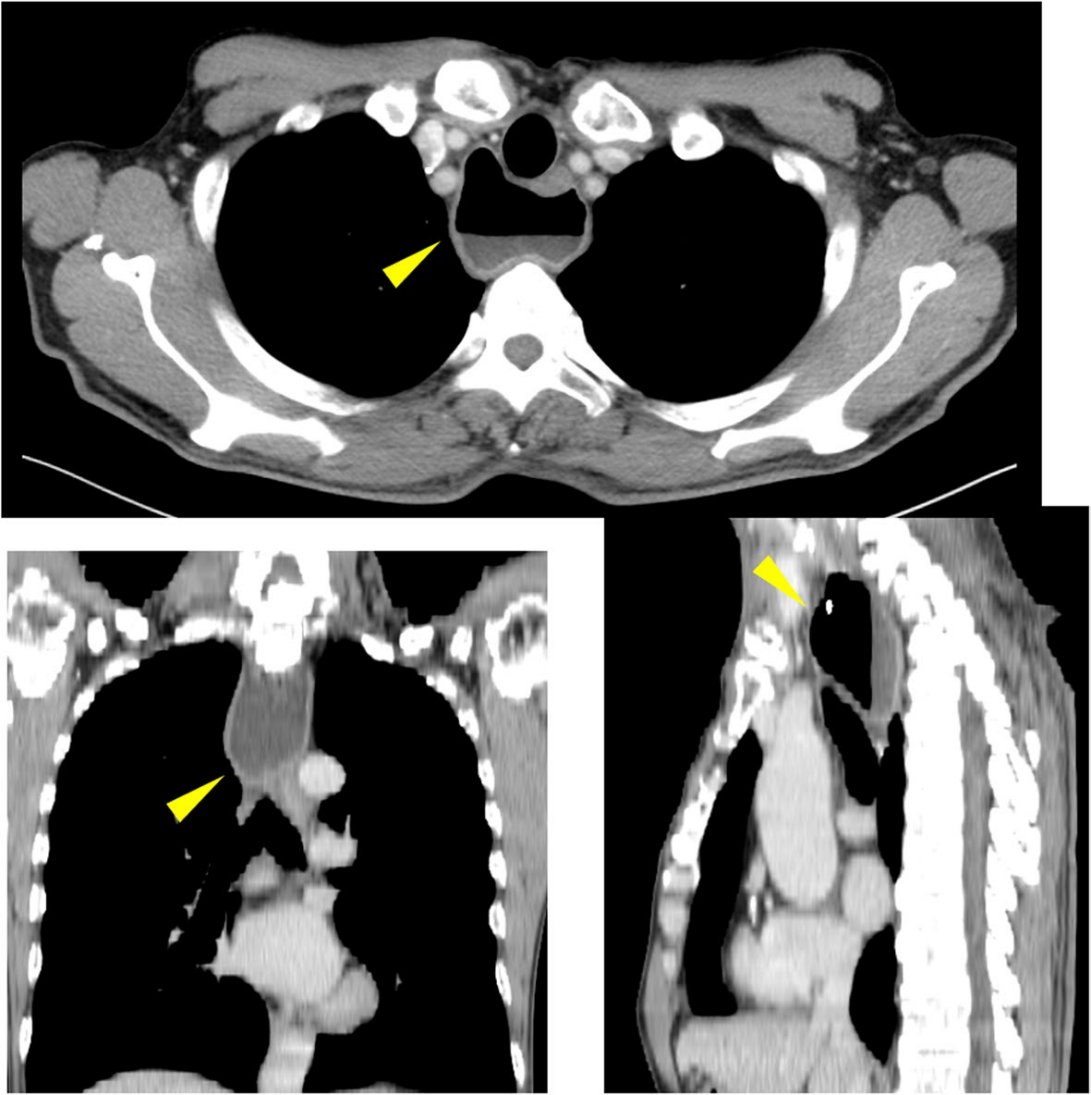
Chest contrast-enhanced CT scan. A diverticulum was observed in the cervical esophagus with fluid retention inside. Tumor localization is unclear
